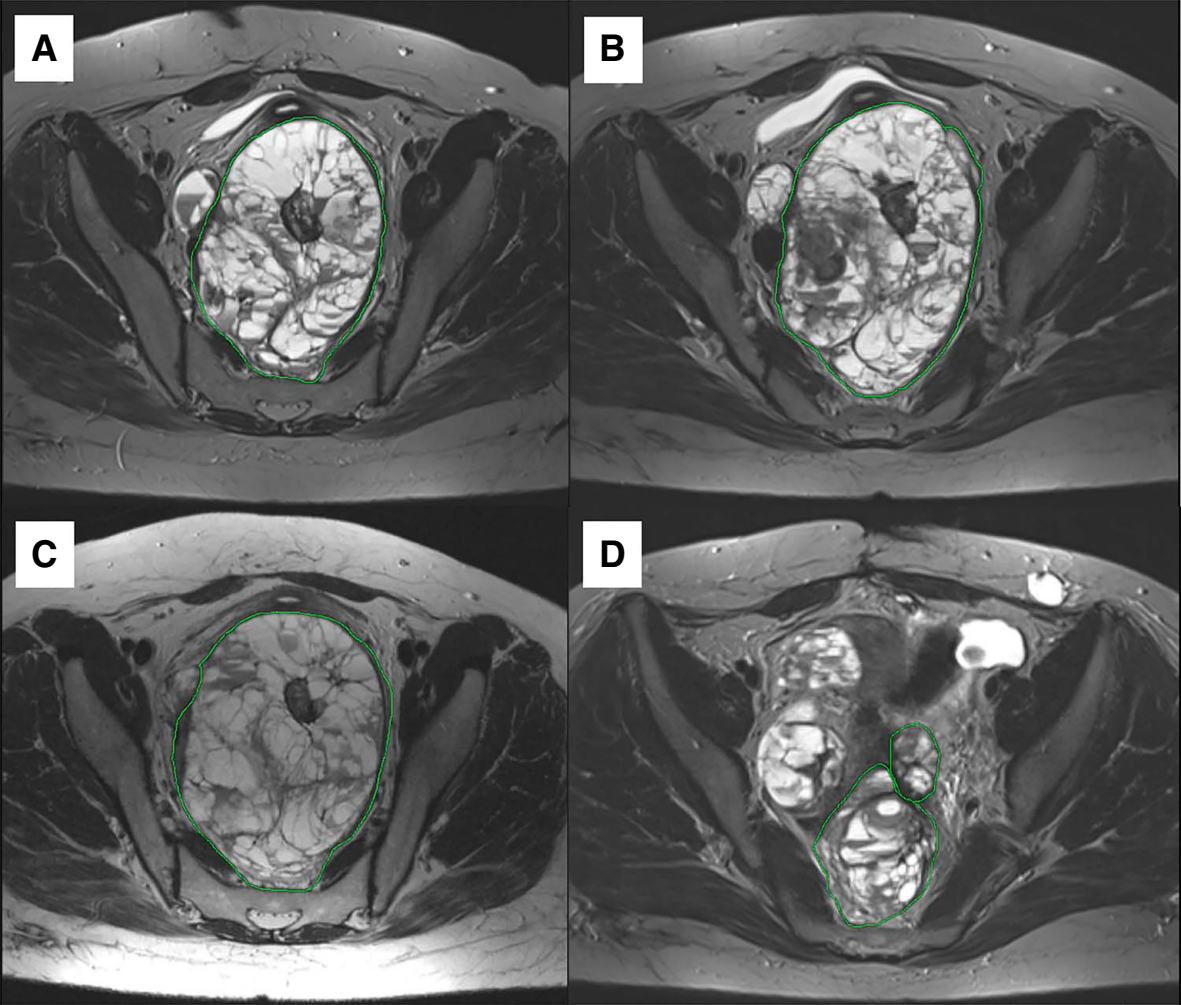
Cystic-solid multinodular formation in Douglas space, part of nodules with high-density content (Neoplasm #2). (*А*) MRI from 03.2016; (*B*) MRI from 06.2016; (*C*) MRI from 09.2016; (*D*) MRI from 03.2018.
